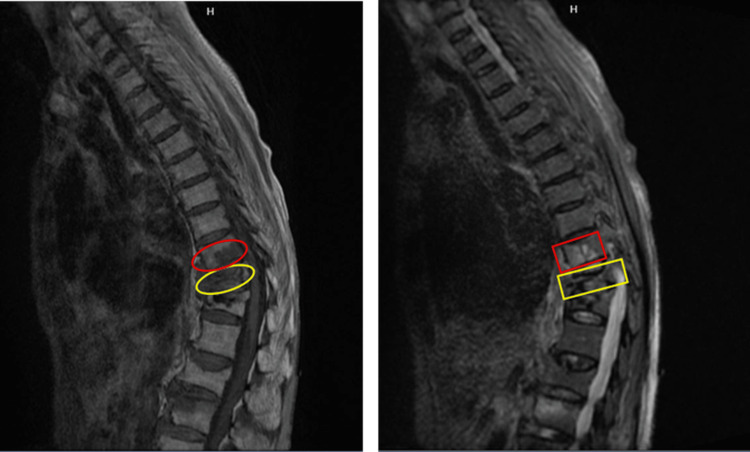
MRI of the thoracic spine without contrast: T1-weighted (left) and STIR (right) images. Red marks indicate T10 changes consistent with a compression fracture; yellow marks highlight T11 changes with a severe vertebral body fracture. STIR, short tau inversion recovery
